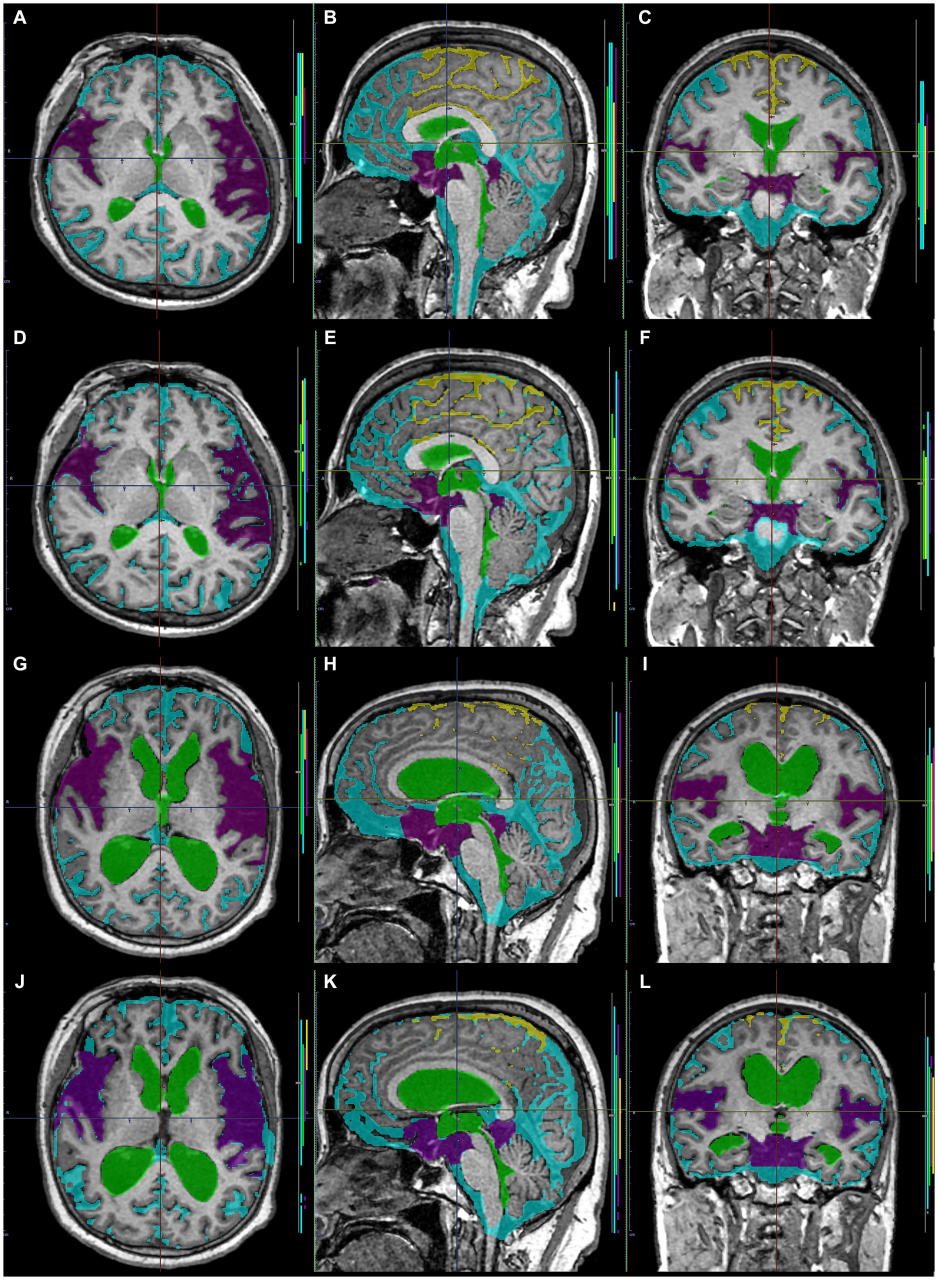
Comparison between manually and automatically segmented regions from 3D T1-weighted images. 3D T1-weighted images in a representative healthy volunteer (A–F) and a representative patient with Hakim disease and DESH (G–L): manually segmented (A–C,G–I) and automatically segmented (D–F,K–L) volumes of the total ventricles (green); Sylvian fissure and basal cistern (purple); high-convexity part of the subarachnoid space (yellow); other subarachnoid spaces (marine blue) from 3D T1-weighted images.
